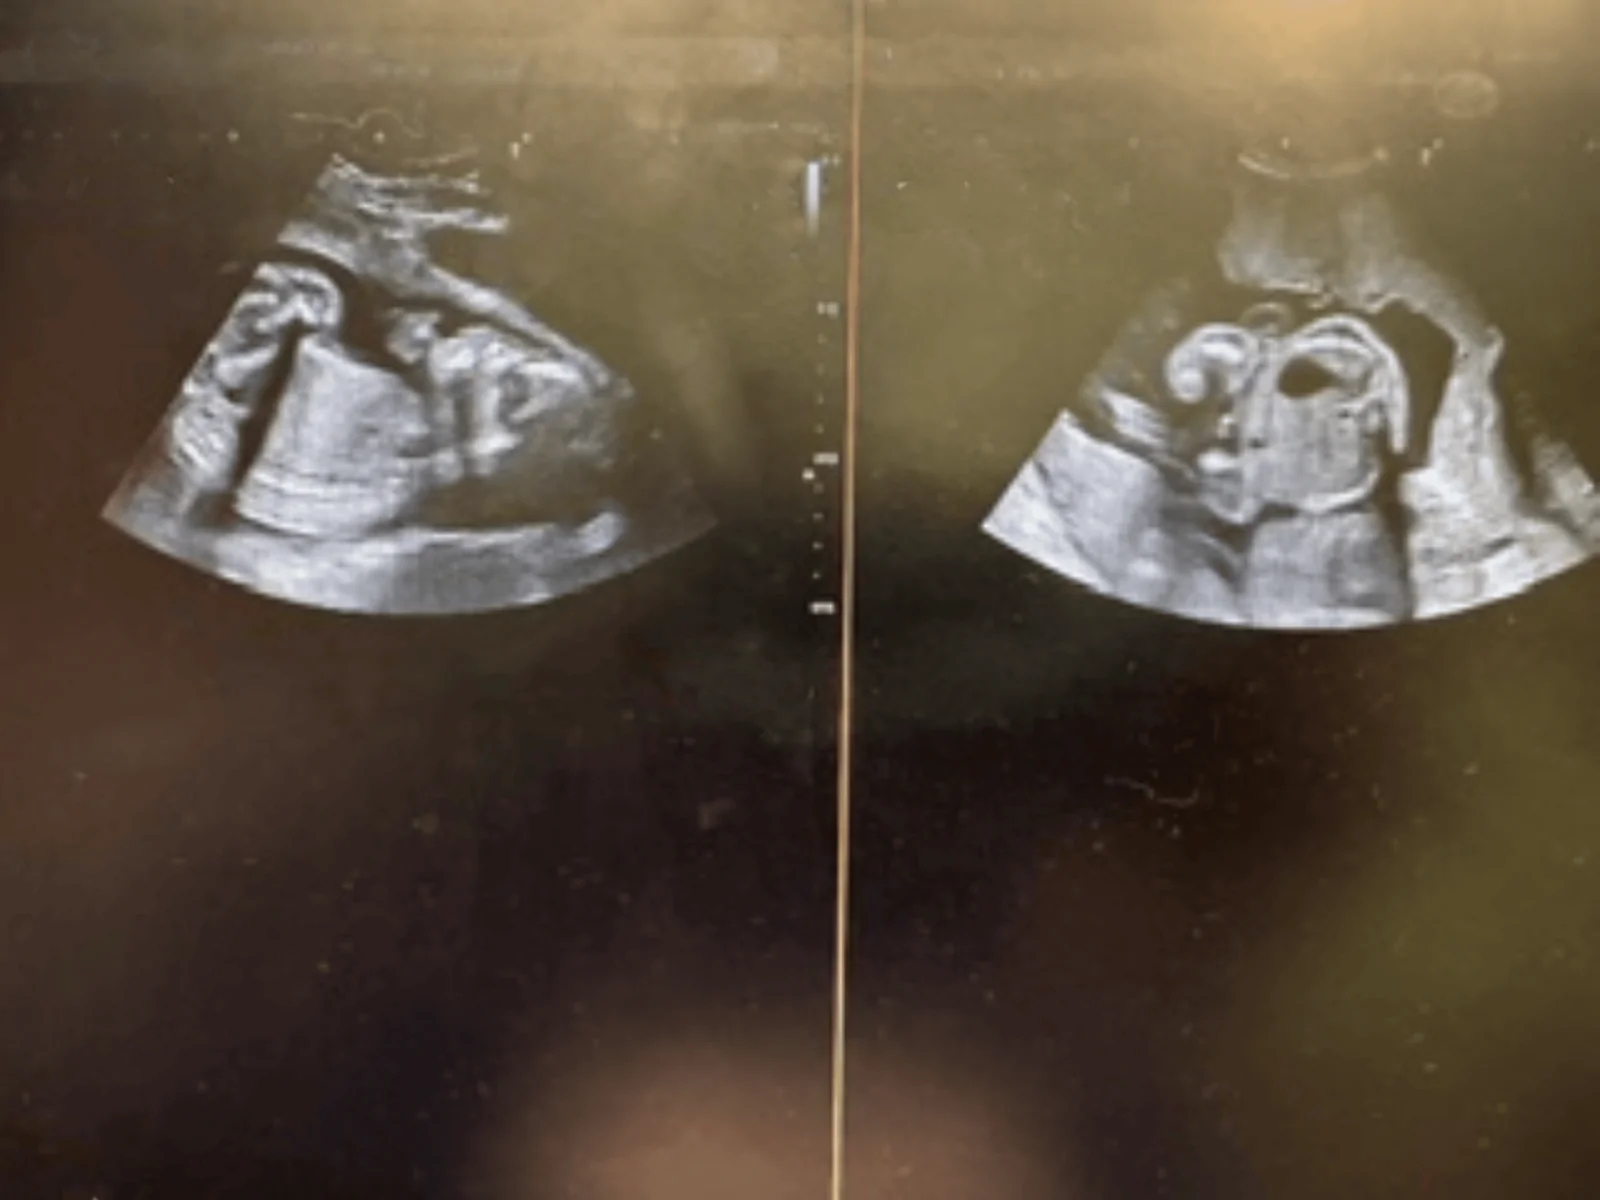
Twin pregnancy dichorionic diamniotic on ultrasound.
